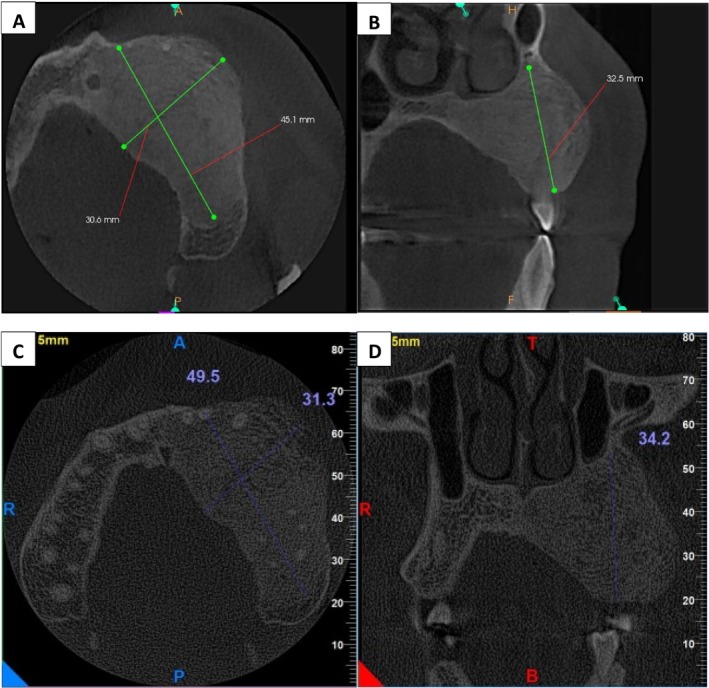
CT scan of the lesion before COVID‐19 infection in two intervals. (A, B) At the time of diagnosis. (C, D) Two years after the diagnosis.
